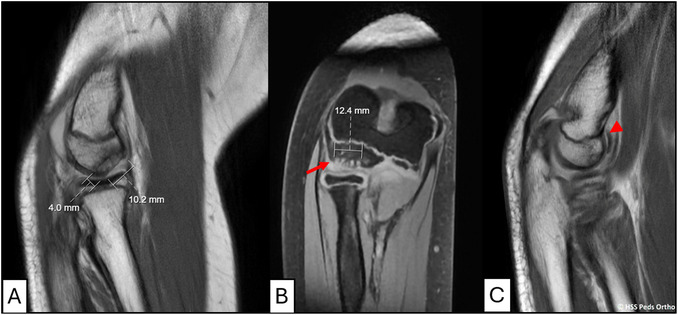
Sagittal proton density MRI sequence (A) displays a 4 mm deep, 10 mm anterior to posterior OCD lesion of the capitellum extending to the 3 o'clock position proximally. A fast spoiled gradient echo fat‐saturated coronal sequence (B) shows the lesion to be contained with an intact radial border (arrow), measuring approximately 12 mm medial to lateral. This sagittal PD sequence (C) shows an approximately 1 × 1 cm loose body in the anterior compartment (arrowhead). (MRI, magnetic resonance imaging; OCD, osteochondritis dissecans; PD, proton density.)
